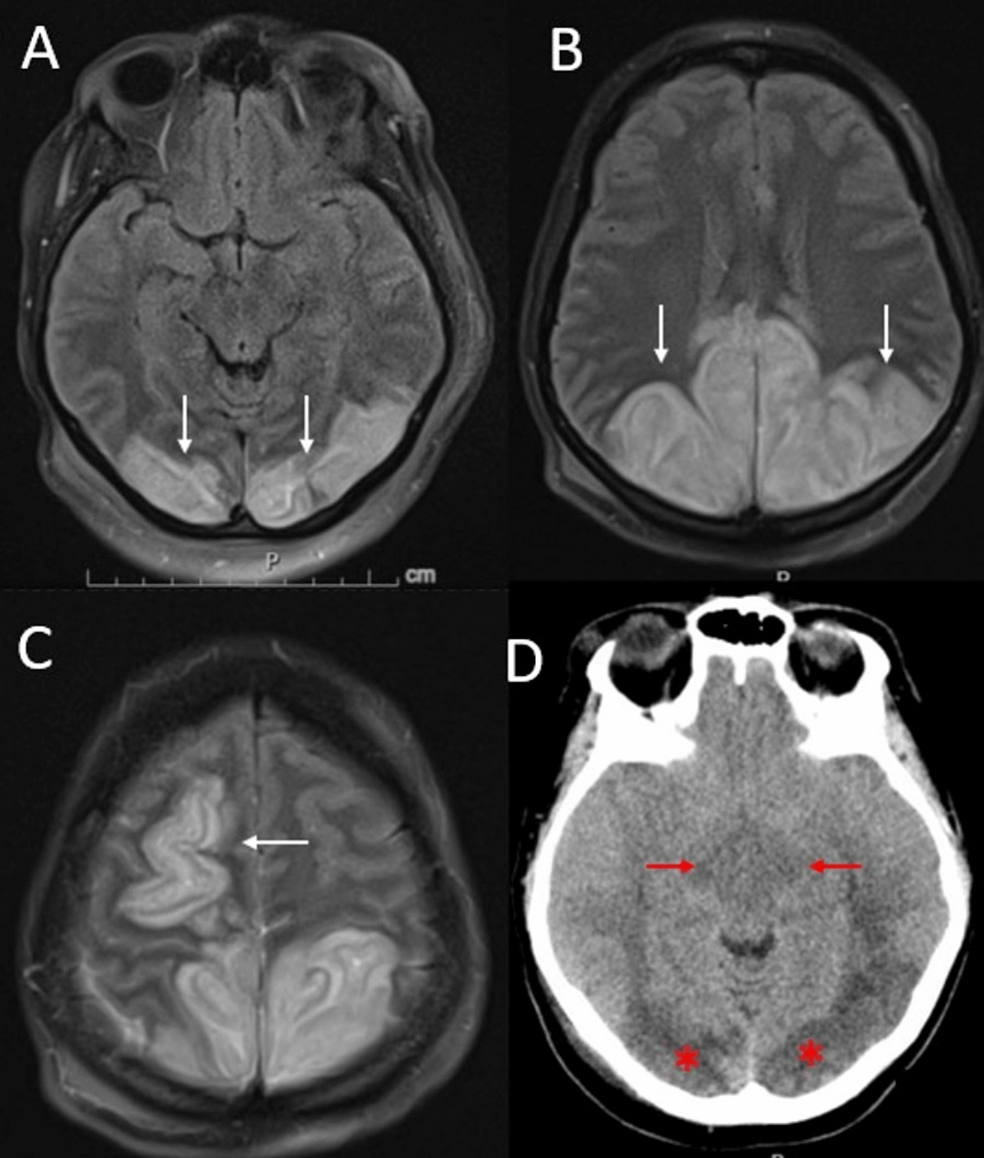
Magnetic resonance imaging and computed tomography of the brain Fluid attenuated inversion recovery sequence demonstrating hyperintensities in subcortical white matter of bilateral occipital (A, white arrows), bilateral parietal (B, white arrows), and right frontal lobes (C, white arrows). A CT on the seventh day of hospitalization demonstrates bilateral mass effect on the midbrain with effacement of ambient cisterns in axial view (D, red arrows) and persistent hypodensity following subcortical white matter of bilateral occipital lobes (D, red asterisks).
